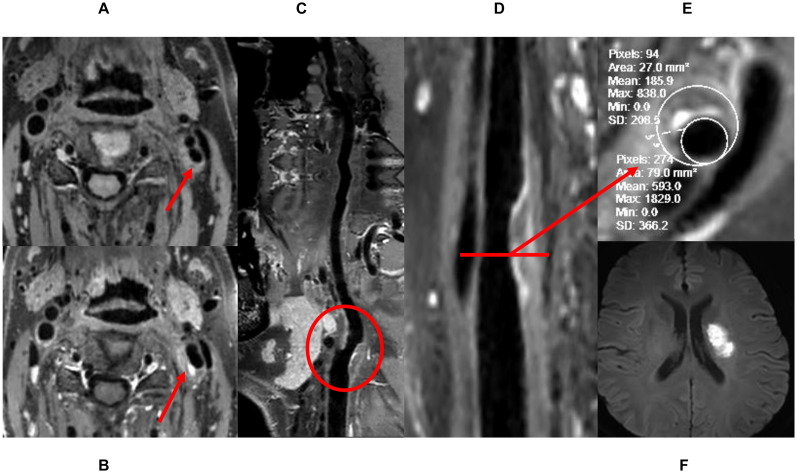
The definition of high-risk plaque. (A) Pre-enhanced HRVW 3D T1 image, inhomogeneous intensity was seen in the plaque. (B) Post-enhanced HRVW 3D T1 image. Hyperintensity was seen in the plaque. (C,D) Reconstruction map of post-enhanced HRVW 3D T1 image. The whole vessel and a plaque shown in red circle were better displayed. (E) Section image perpendicular to the lumen in the plaque lesion. Stenosis rate was determined in this section. (F) DWI. Hyperintensity was seen in left basal ganglia, indicating cerebrovascular events occurred.
